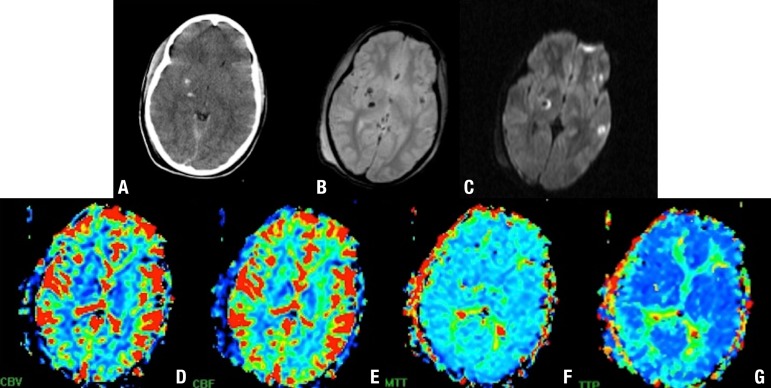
DAI involving the basal ganglia and gray-white matter junction. Axial CT
